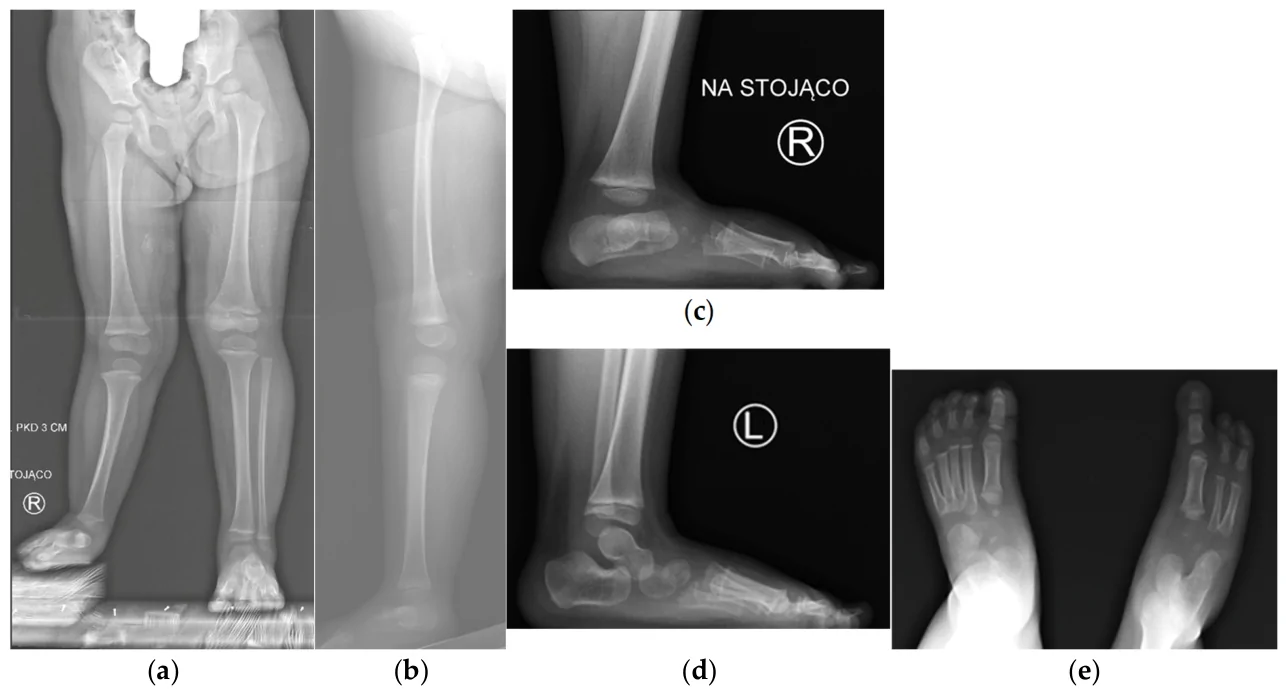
Radiographic views in fibular hemimelia: (a) full-length standing anteroposterior radiograph showing tibial shortening, valgus mechanical axis, ankle valgus; (b) lateral standing radiograph of the right limb with normal knee orientation; (c) lateral standing radiograph of the right foot demonstrating valgus alignment between the calcaneus and talus and talocalcaneal coalition; (d) lateral standing radiograph of a right foot (normal); (e) standing weight-bearing anteroposterior foot radiograph showing talocalcaneal coalition with valgus deformity and absence/hypoplasia of the lateral rays of the right foot. L—left, R—right, PKD—right lower limb, NA STOJĄCO—standing position.
